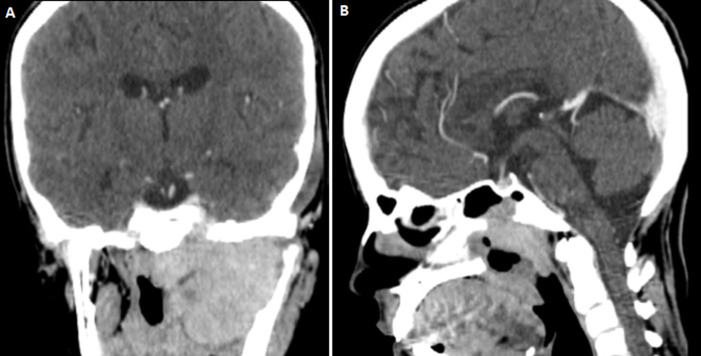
A) cervical CT scan with sagittal reconstruction showing the endocranial extension of the tumour through the carotid canal; B) with the lysis of the floor of the sphenoidal sinus
